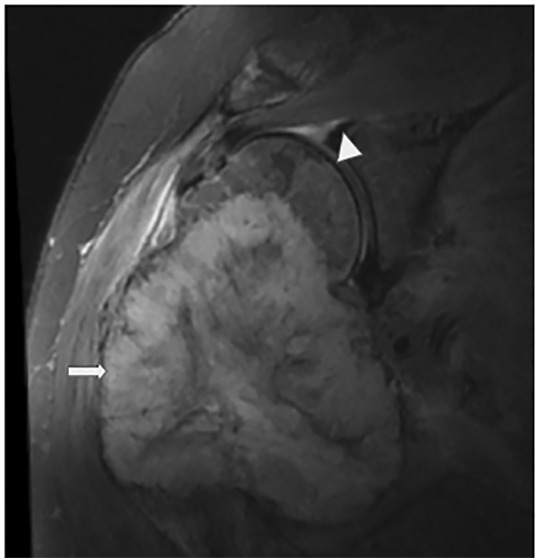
Magnetic resonance imaging of the right humerus demonstrates a large, heterogeneous mass (arrow) adjacent to the right humeral neck and head, which extends peripherally into the soft tissues, representing malignancy. Arrowhead denotes the normal humeral head.
